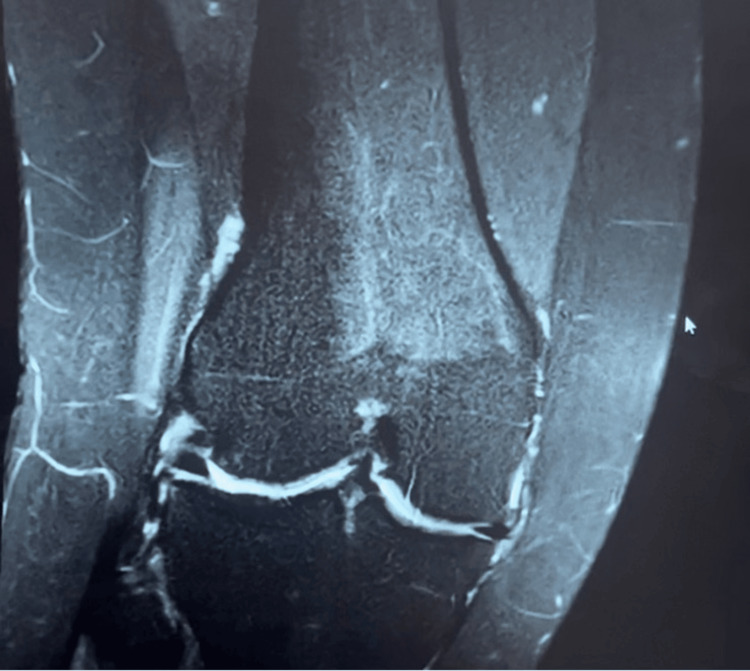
Coronal MRI of the right knee demonstrating lateral compartment joint space narrowing MRI: magnetic resonance imaging
